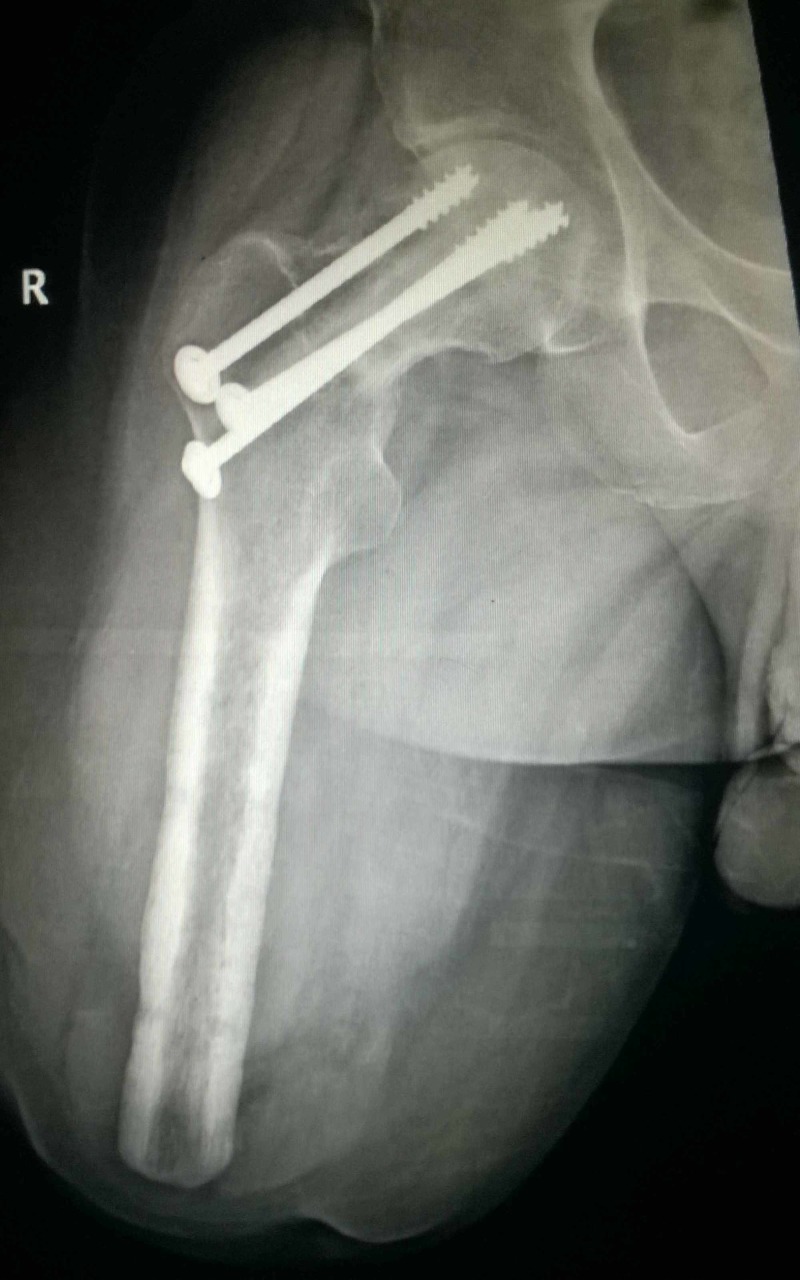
One and half years follow up antero-posterior radiograph of patient A showing the incorporation of fibula and healed fracture
